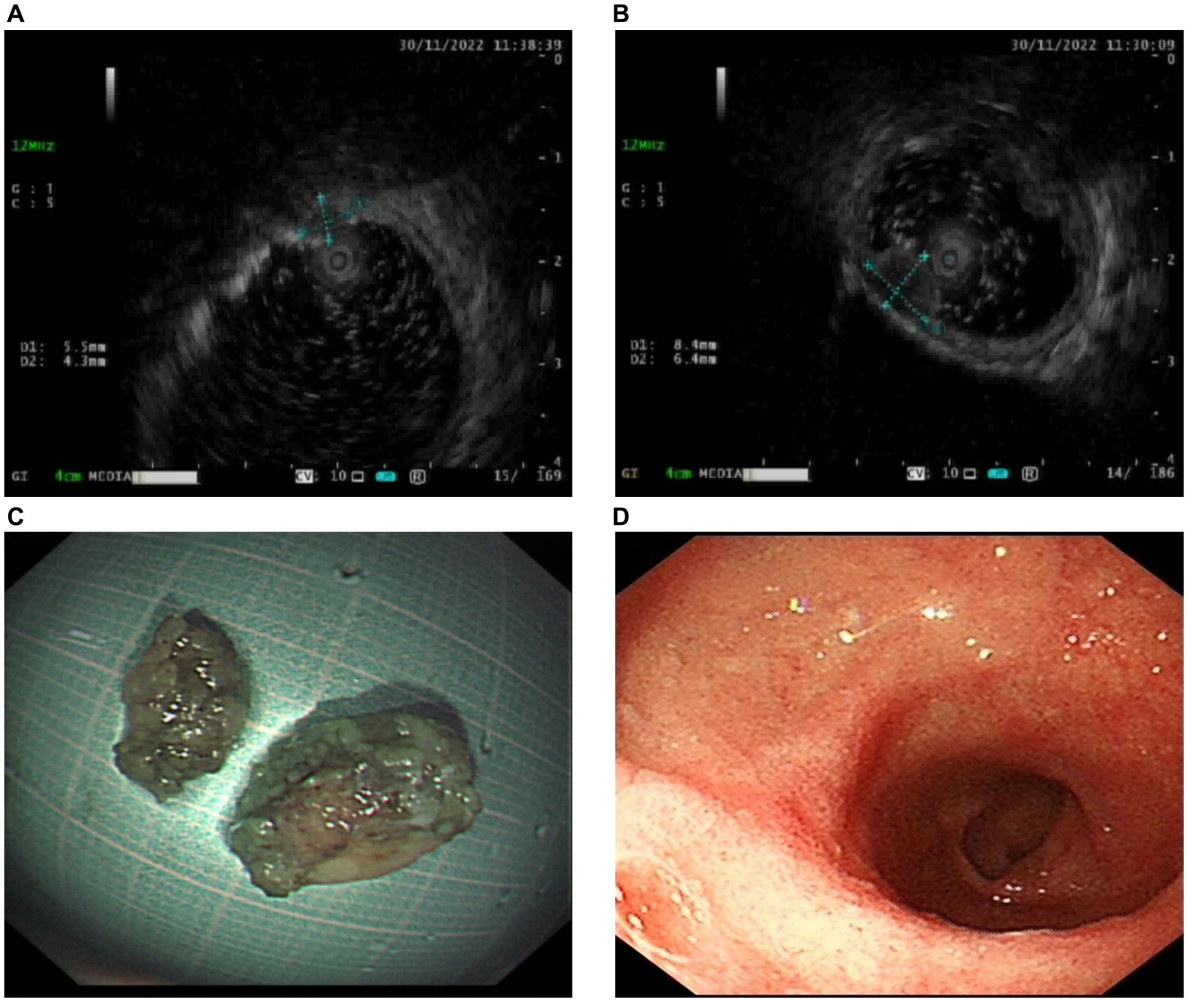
Endoscopic ultrasonography and resected lesions. (A,B) Endoscopic ultrasonography shows two hypoechoic lesions (A measuring 5.5*4.3 mm and B measuring 8.4*6.4 mm) originating from muscularis propria of the duodenal bulb. (C) The resected lesions were pulled out. (D) Postoperative gastroscopy 3 months later showed the healing of the resection sites.
